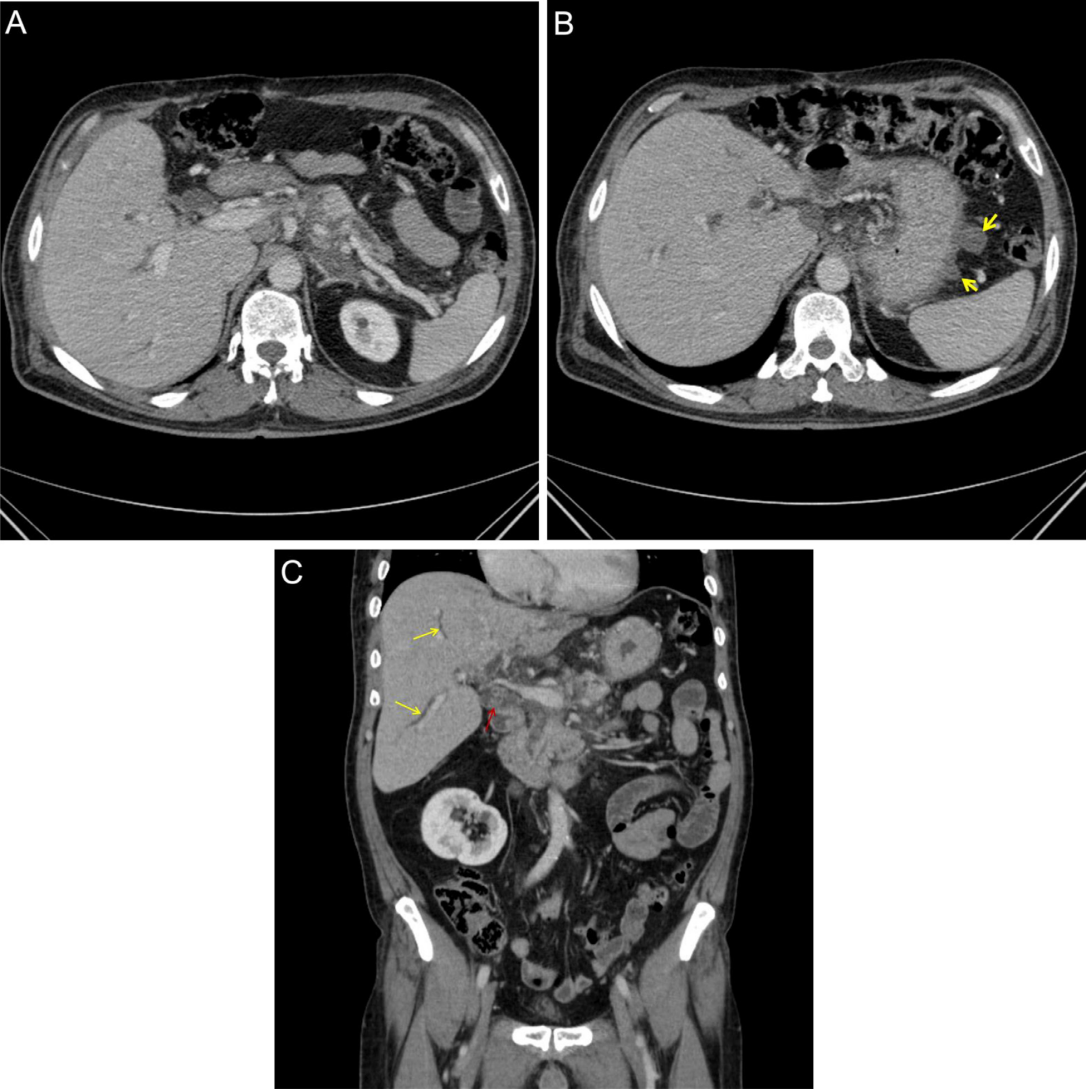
(A-C) Abdomen and pelvic CT scan, Axial and Coronal views: (A) The head and tail of the pancreas show normal size with mild inhomogeneous parenchymal density. The body of the pancreas is not seen due to previous resection. No evidence of pancreatic pseudocyst is seen. (B) Evidence of 2 newly cystic structures measuring up to 20 × 19 mm is seen in the greater curvature of the stomach. According to the patient's medical history, this is suggestive of an intramural gastric pseudocyst (2 yellow arrows). (C) Multiple stones are seen in the common bile duct (CBD) (red arrow), associated with dilatation of the common bile duct (red arrow) and intrahepatic bile ducts (yellow arrows) as the cause of recurrent pancreatitis.
